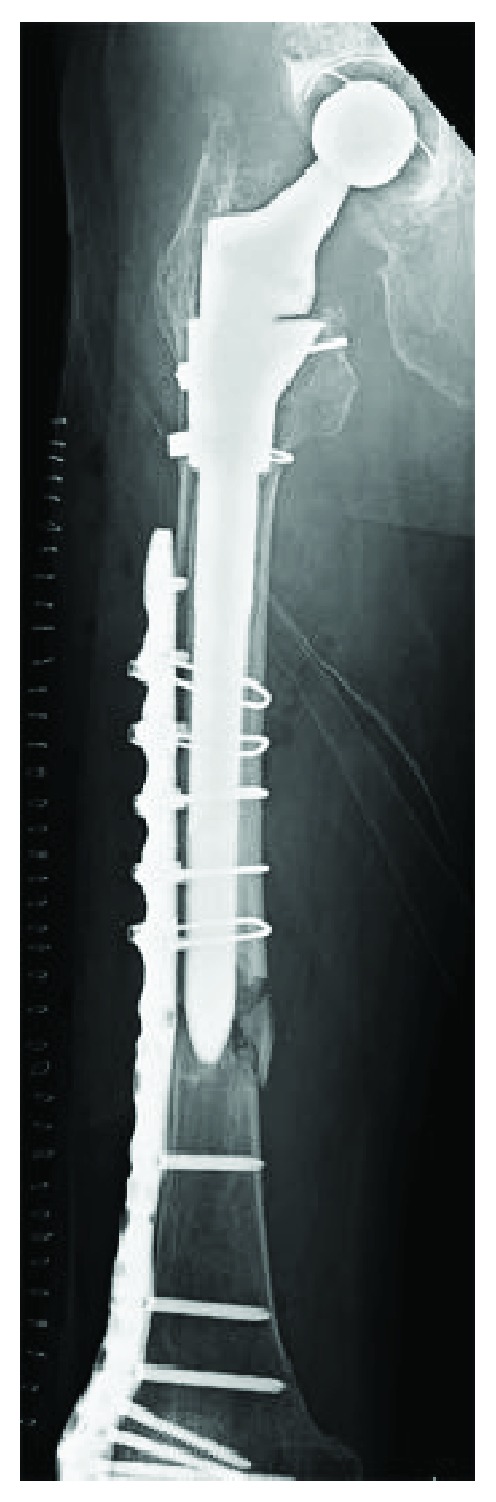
Anteroposterior radiograph showed fixation of the periprosthetic fracture using a cable-plate device following the operation.
